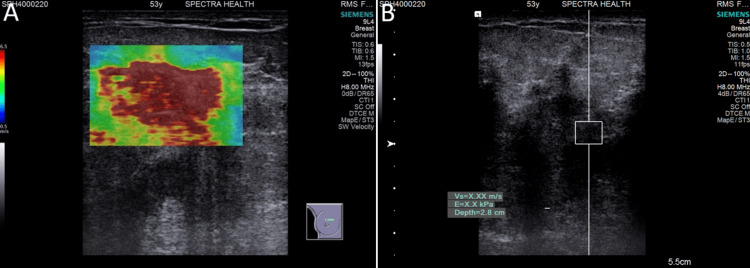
Malignant lesion: (A) Qualitative elastography shows very high stiffness with a predominantly red color map. (B) Quantitative elastography shows very high stiffness with acoustic radiation force impulse (ARFI) velocity beyond the measurable range recorded as X.XX m/s (≥ 6 m/s). X.XX m/s denotes SWE exceeding the default upper limit of ultrasound scanner (≥6 m/s).
